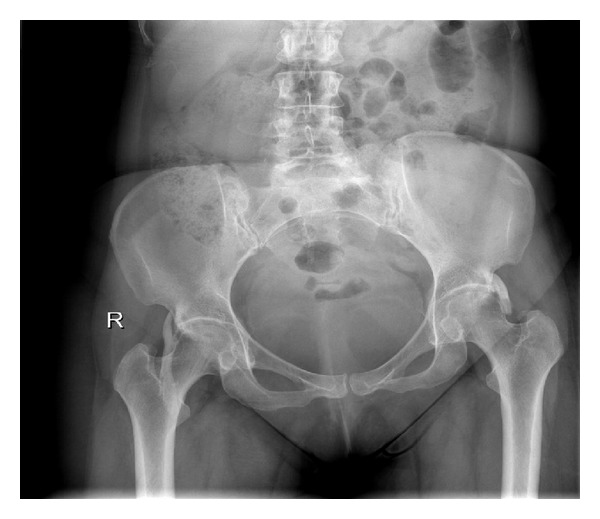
Anteroposterior X-ray of the pelvis at the initial presentation.
